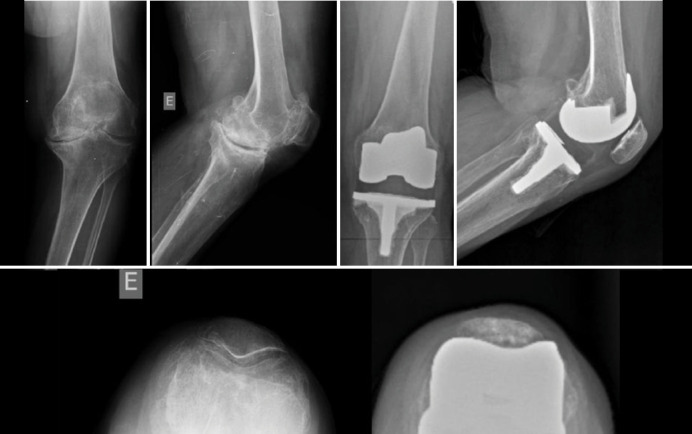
X-ray analysis with 2 years of implant evolution with constricted polyethylene.
